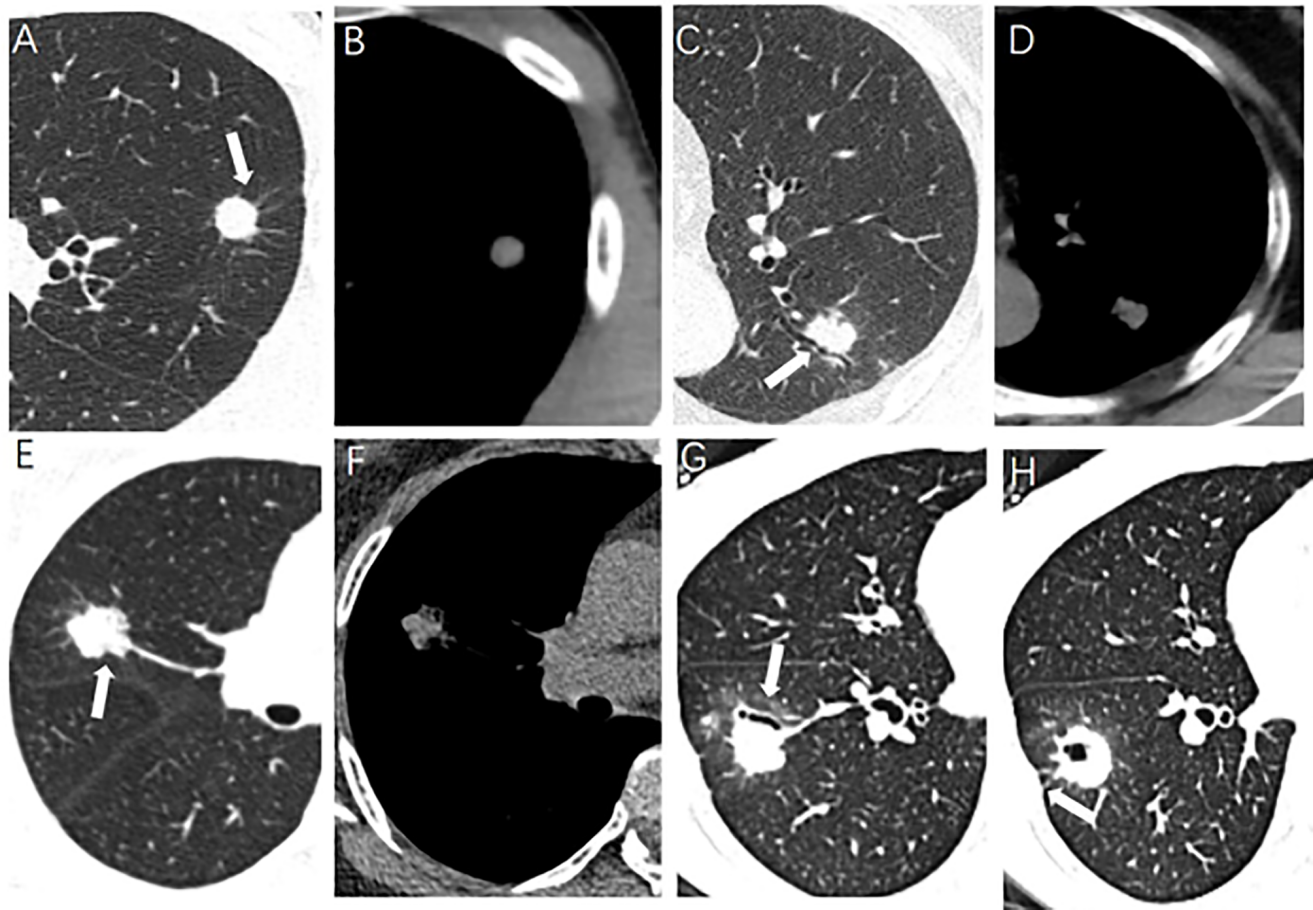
Some CT morphological characteristics of pulmonary solitary solid nodule. (A, B) A 30-year-old male, lung tuberculoma (LTB) with spiculated sign (arrow). (C, D) A 65-year-old female, LTB with air bronchogram (arrow). (E, F) A 62-year-old female, lung adenocarcinoma (LAC) with lobulated sign (arrow). (G, H) A 33-year-old female, LAC with air bronchogram and pleural indentation (arrow).
